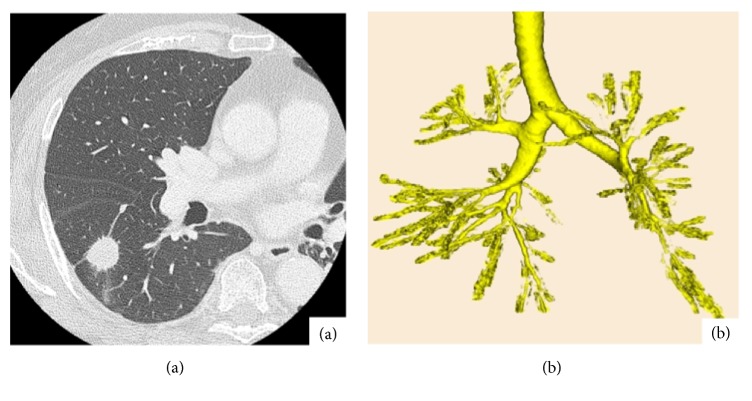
(a) Chest computed tomography (CT) showing a 1.8 cm, irregular nodular lesion in the right lower pulmonary lobe. (b) Three-dimensional CT showing normal bronchial anatomy.
